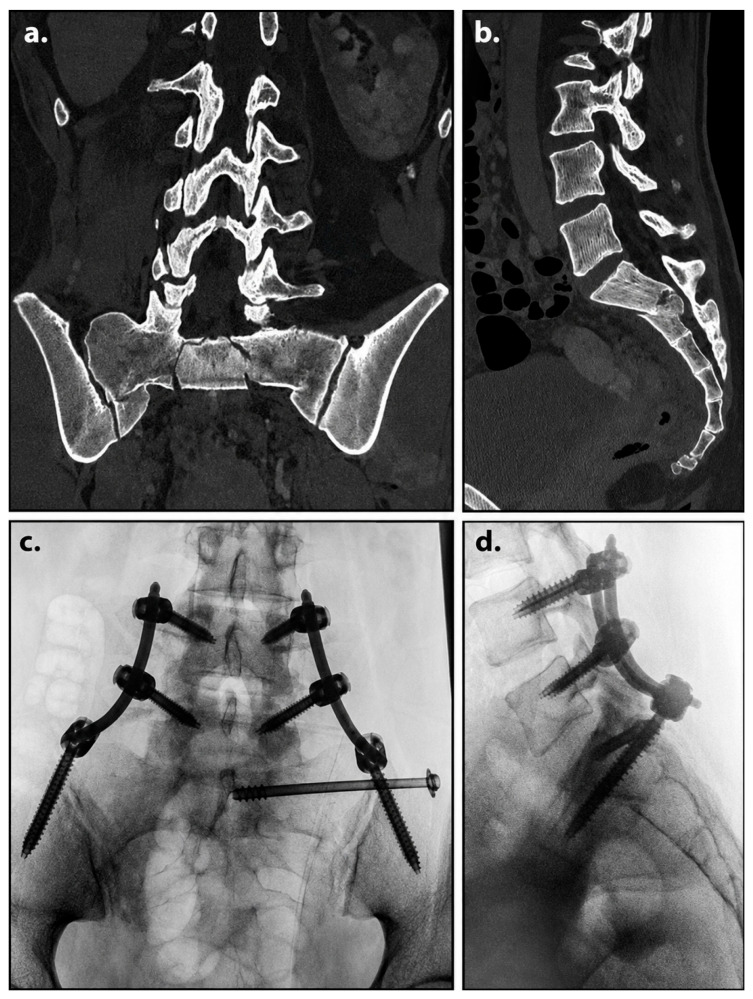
Case 1: Preoperative and postoperative imaging of Case 1. (a) Coronal CT reconstruction showing a U-shaped sacral fracture with lumbopelvic dissociation. (b) Sagittal CT reconstruction demonstrating kyphotic angulation of approximately 45° at S2 with involvement of the L5–S1 facet joints. (c) Postoperative anteroposterior radiograph after closed reduction and percutaneous fixation, showing bilateral pedicle screws at L5 and iliac screws connected by rods with a trans-sacral screw. (d) Postoperative lateral radiograph confirming restoration of alignment and stable construct positioning.
